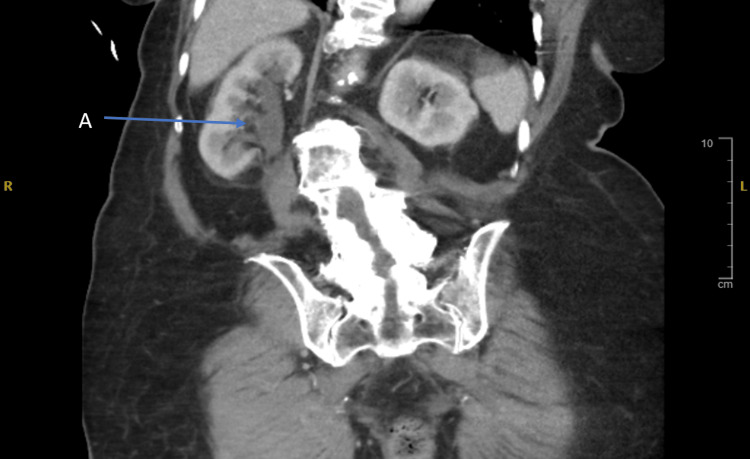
Coronal view of contrasted computed tomography of the abdomen and pelvis, which showed right-sided hydronephrosis (A).
